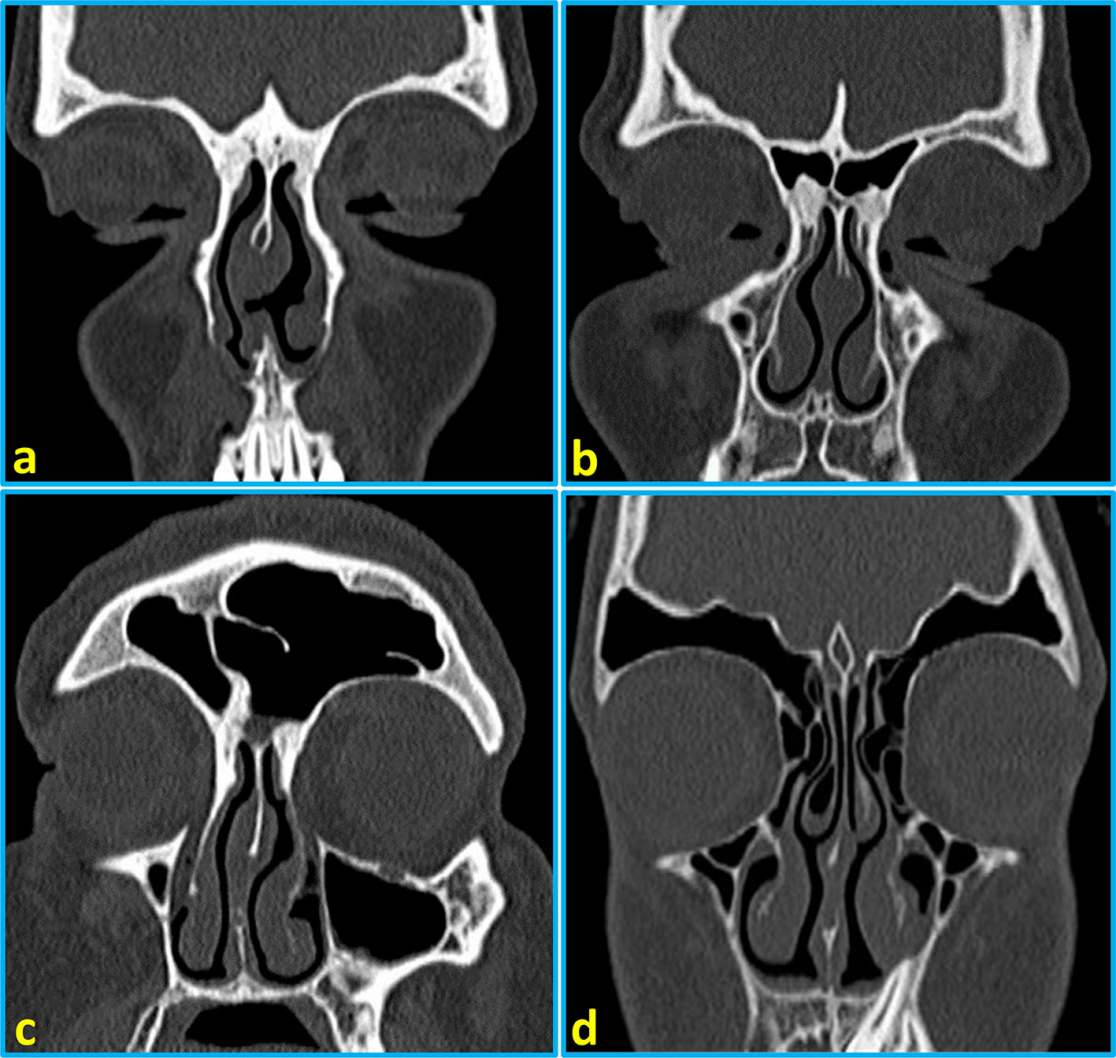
Reformatted coronal computed tomography images of the paranasal sinuses showing types of frontal sinus pneumatization: (a) bilateral aplasia of the frontal sinuses, (b) bilateral hypoplasia, (c) medium-sized pneumatization of the right frontal sinus and hyperplasia of the left frontal sinus, and (d) bilateral hyperplasia of the frontal sinuses.
